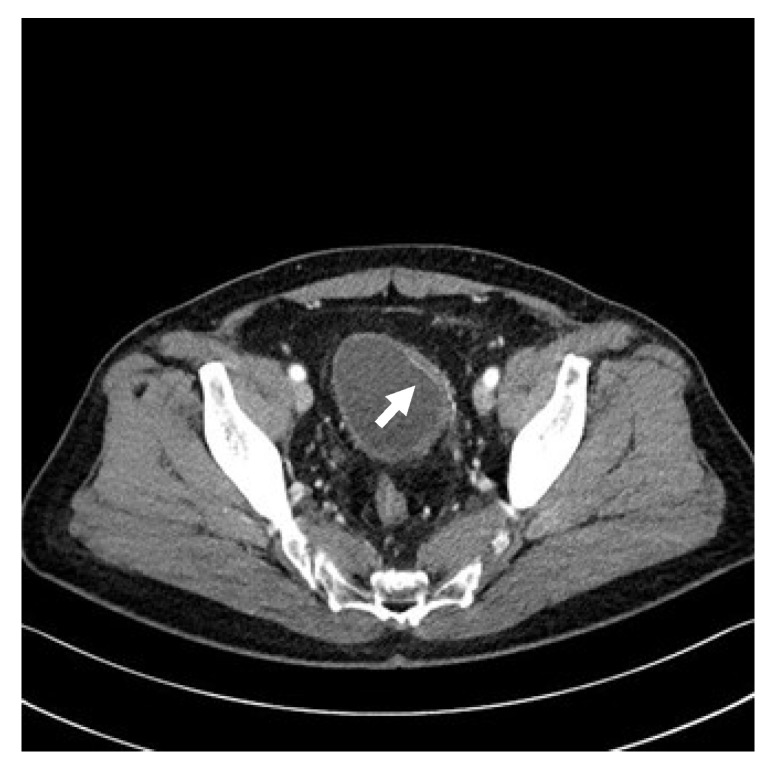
Pelvic computed tomography. Pelvic computed tomography reveals a mild bladder wall thickening with prominent mucosal enhancement (white arrow).
